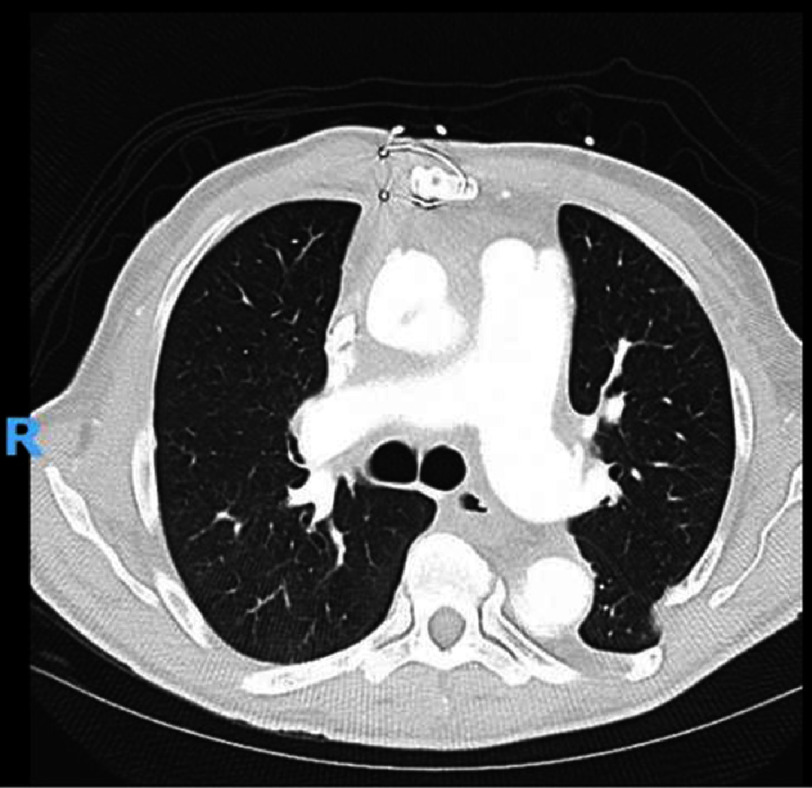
Axial Image of a postoperative CT scan of a patient following the repair of an aortic pseudoaneurysm at the distal suture line of a prior aortic arch replacement on VV ECMO. Axial image of the chest at the level of T7 in a patient following pseudoaneurysm repair 10 months after ascending and aortic arch replacement for acute type A aortic dissection. Mediastinal anatomy and trachea are consistent with resolution of the large pseudoaneurysm. Abbreviations: CT is computed tomography, VV ECMO is venovenous extracorporeal membrane oxygenation.
